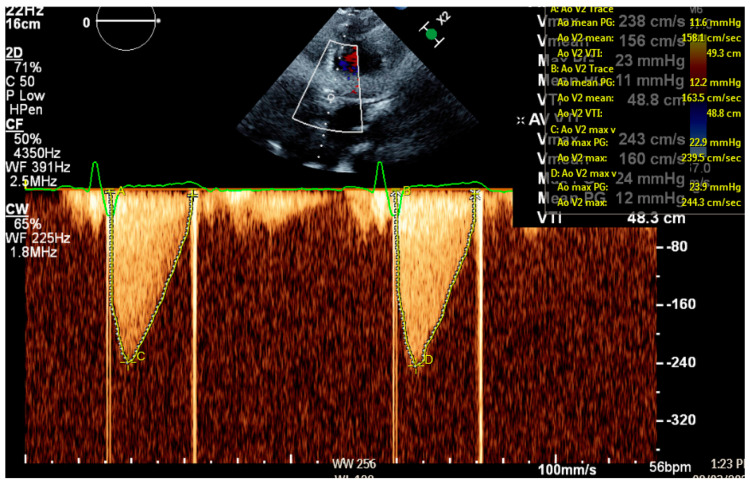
Transthoracic echocardiography following ultraslow low-dose alteplase infusion showing a mean aortic transvalvular gradient of 12 mmHg and aortic valve peak velocity of 283 cm/s, indicating resolution of prosthetic valve thrombosis.
